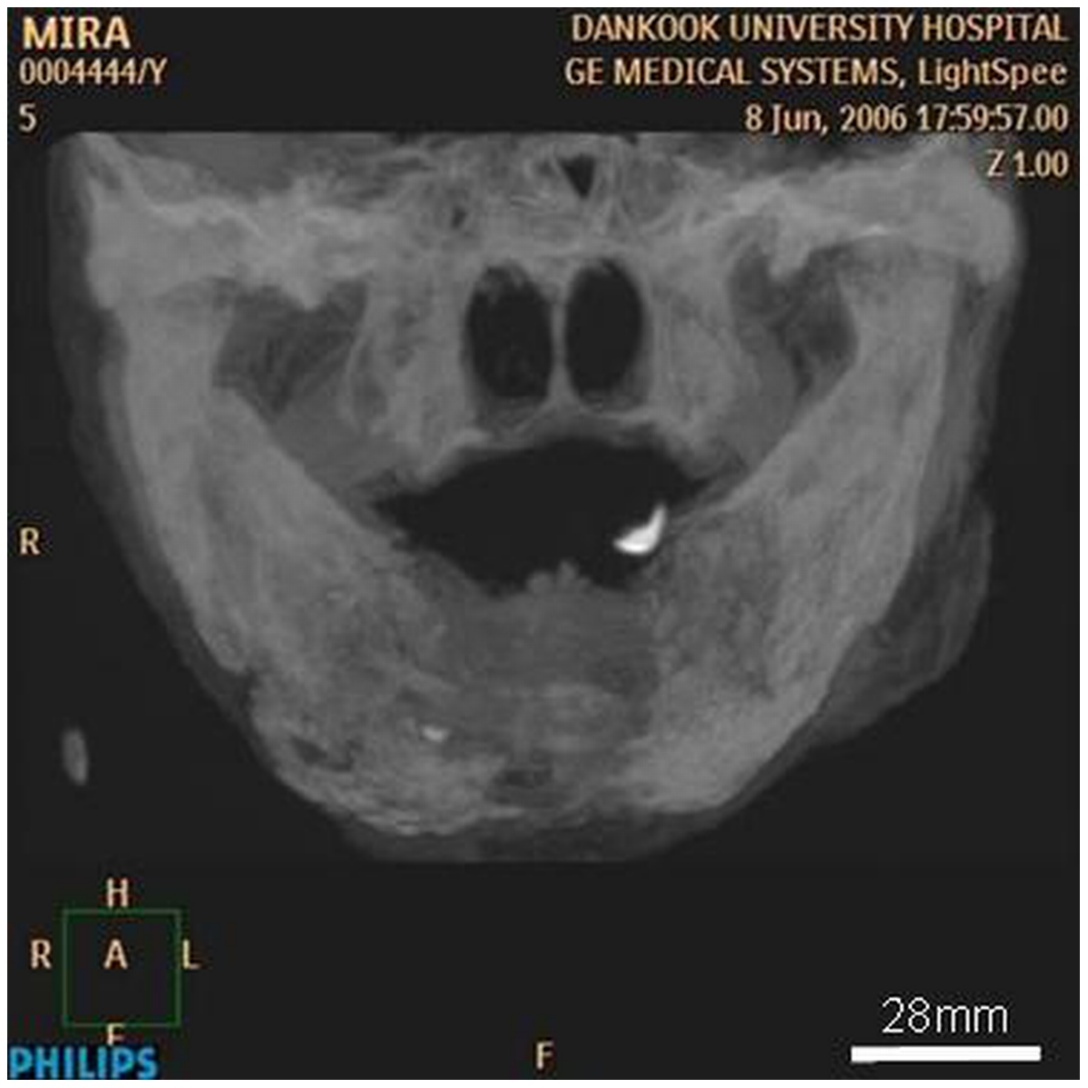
Radiographic image of the jaws demonstrates heterogeneous sclerosis of the bone, corresponding to the classic “ground-glass” appearance. Note the radiolucent shadow at the mental area.
